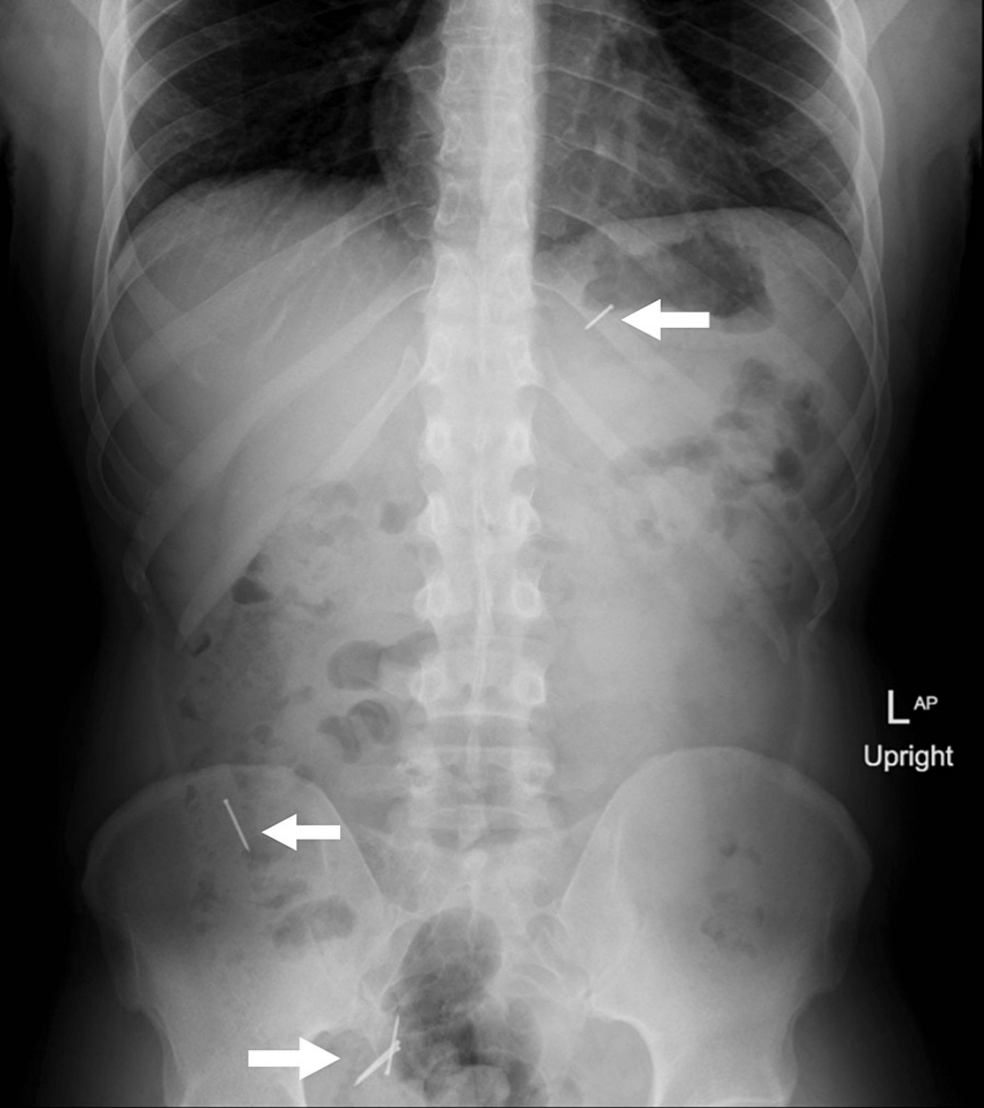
Abdominal X-ray showing five nails.
